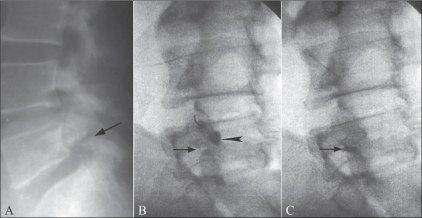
Facet injection in spondylolysis. The lateral radiograph (A) shows a break in the pars interarticularis (arrow). Oblique fluoroscopic spot image (B) shows a fluoroscopy-guided L4–5 facet injection with filling of the inferior recess (arrowhead). Note the spondylolysis (arrow). A more delayed image (C) shows tracking of contrast from the facet joint into the spondylolytic site (arrow). Note the emptying of contrast from the joint space.
